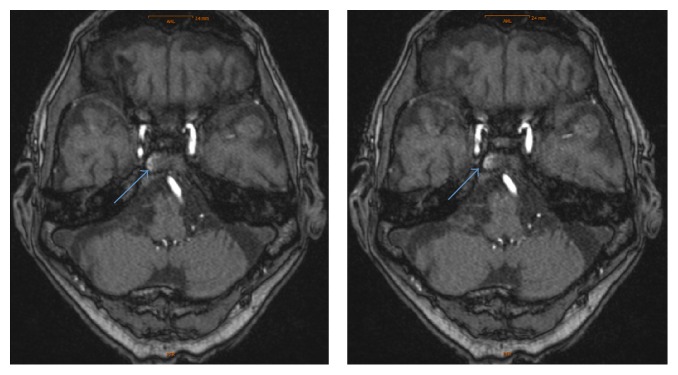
MRI axial images with contrast enhancement of the right cavernous sinus.
